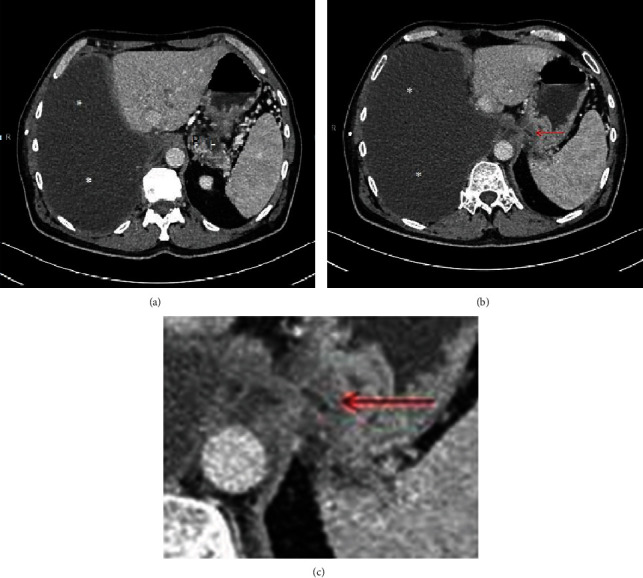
Computed tomography of the abdomen after intravenous contrast injection in axial plane. Enhancement of fistulous track walls that connect peripancreatic fluid collection with right pleural cavity (a, b). Fistula is better seen after enlargement (c). P: pancreas; F: fluid collection. ^∗^Free fluid in pleural cavity. Red arrow: fistula.
